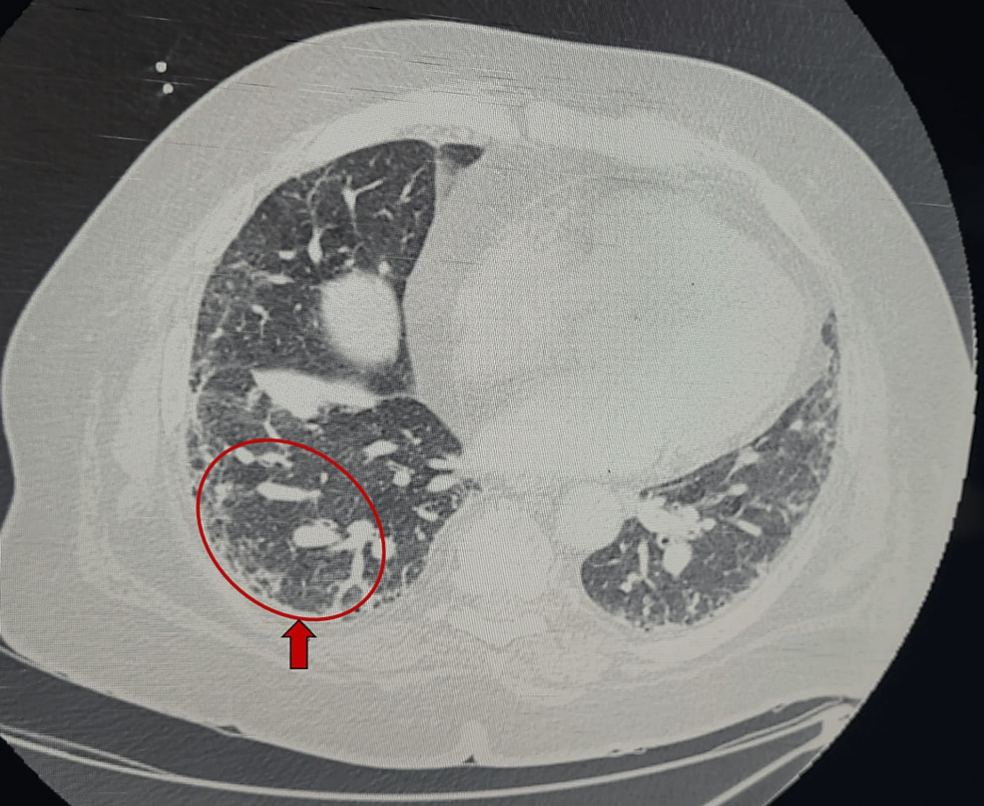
Chest CT scan of the patient showing peripheral honeycombing (red arrow and circle) consistent with pulmonary fibrosis.
